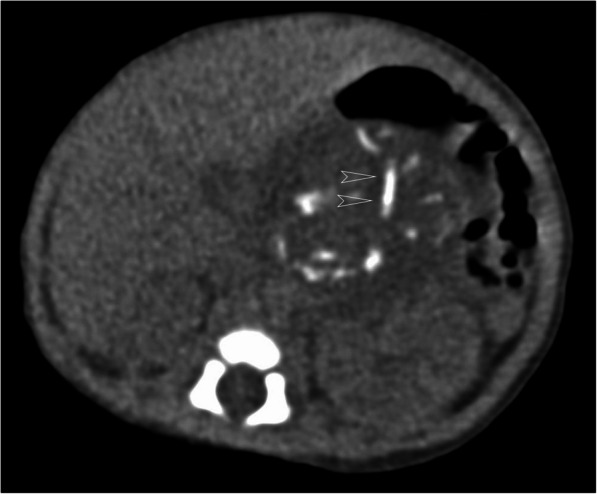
Axial unenhanced CT image of the mass: CT showed a hyperattenuating axial skeleton (arrowhead)
